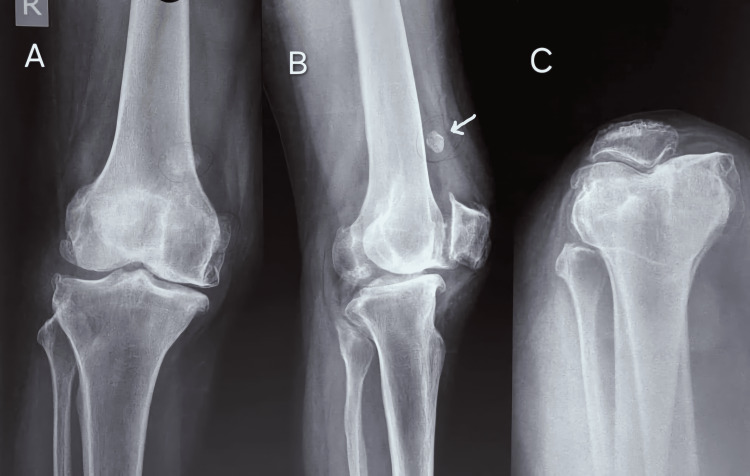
Anteroposterior (A,C) and lateral (B) views of the patient’s knee on a plain radiograph showing a radio-opaque shadow of an intra-articular loose body (white arrow)
